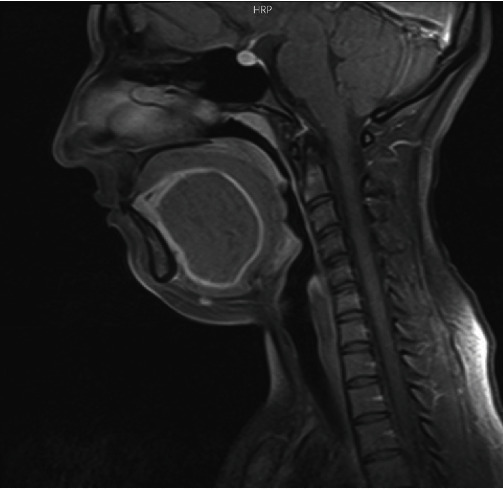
MRI showing a well-defined mass in the sublingual area.
